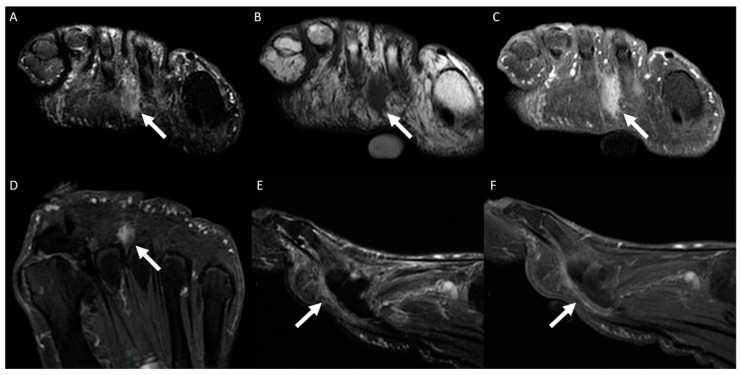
A 77-year-old man presented with left forefoot plantar pain persisting for 30 days. MRI images of the forefoot in short-axis plane, including a T2-weighted fat-suppressed scan (A) and a T1-weighted scan (B), as well as post-gadolinium T1-weighted fat-suppressed scan (C), along with a coronal T2-weighted fat-suppressed scan (D) and a sagittal T2-weighted fat-suppressed scan (E) and a post-gadolinium T1-weighted fat-suppressed scan (F), revealed thrombosis in the plantar metatarsal vein. This condition was characterized by venous dilation, with intraluminal thrombus and an intravenous filling defect (indicated by arrows), mimicking the diagnosis of Morton’s neuroma. Notably, the sagittal plane images (E,F) clearly depict the extent of thrombus within the metatarsal vein.
